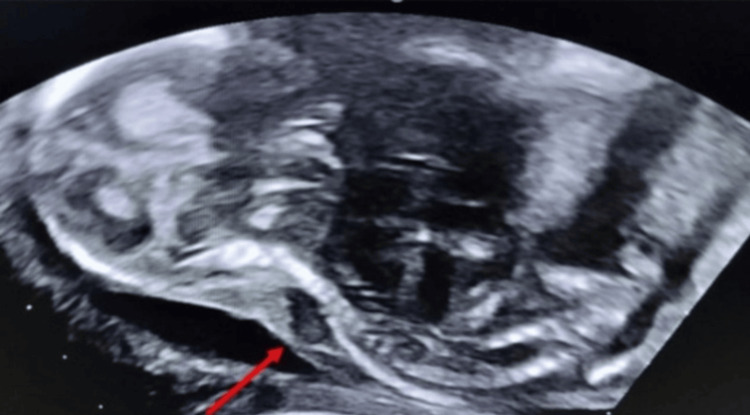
Prenatal ultrasound showing associated skeletal and neck anomalies. B-mode antenatal ultrasound, sagittal view, demonstrating cystic hygroma at the level of the fetal neck and associated kyphoscoliosis of the spine (red arrow).
